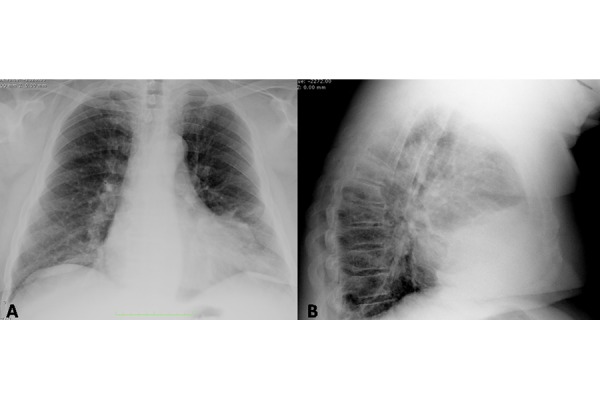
A 72 years-old male affected by polycythemia and COPD. (1A) Postero-anterior and (1B) lateral chest X-Ray show diffuse thickening of peribronchial interstitium. The spine (1B) does not show pathological findings.
